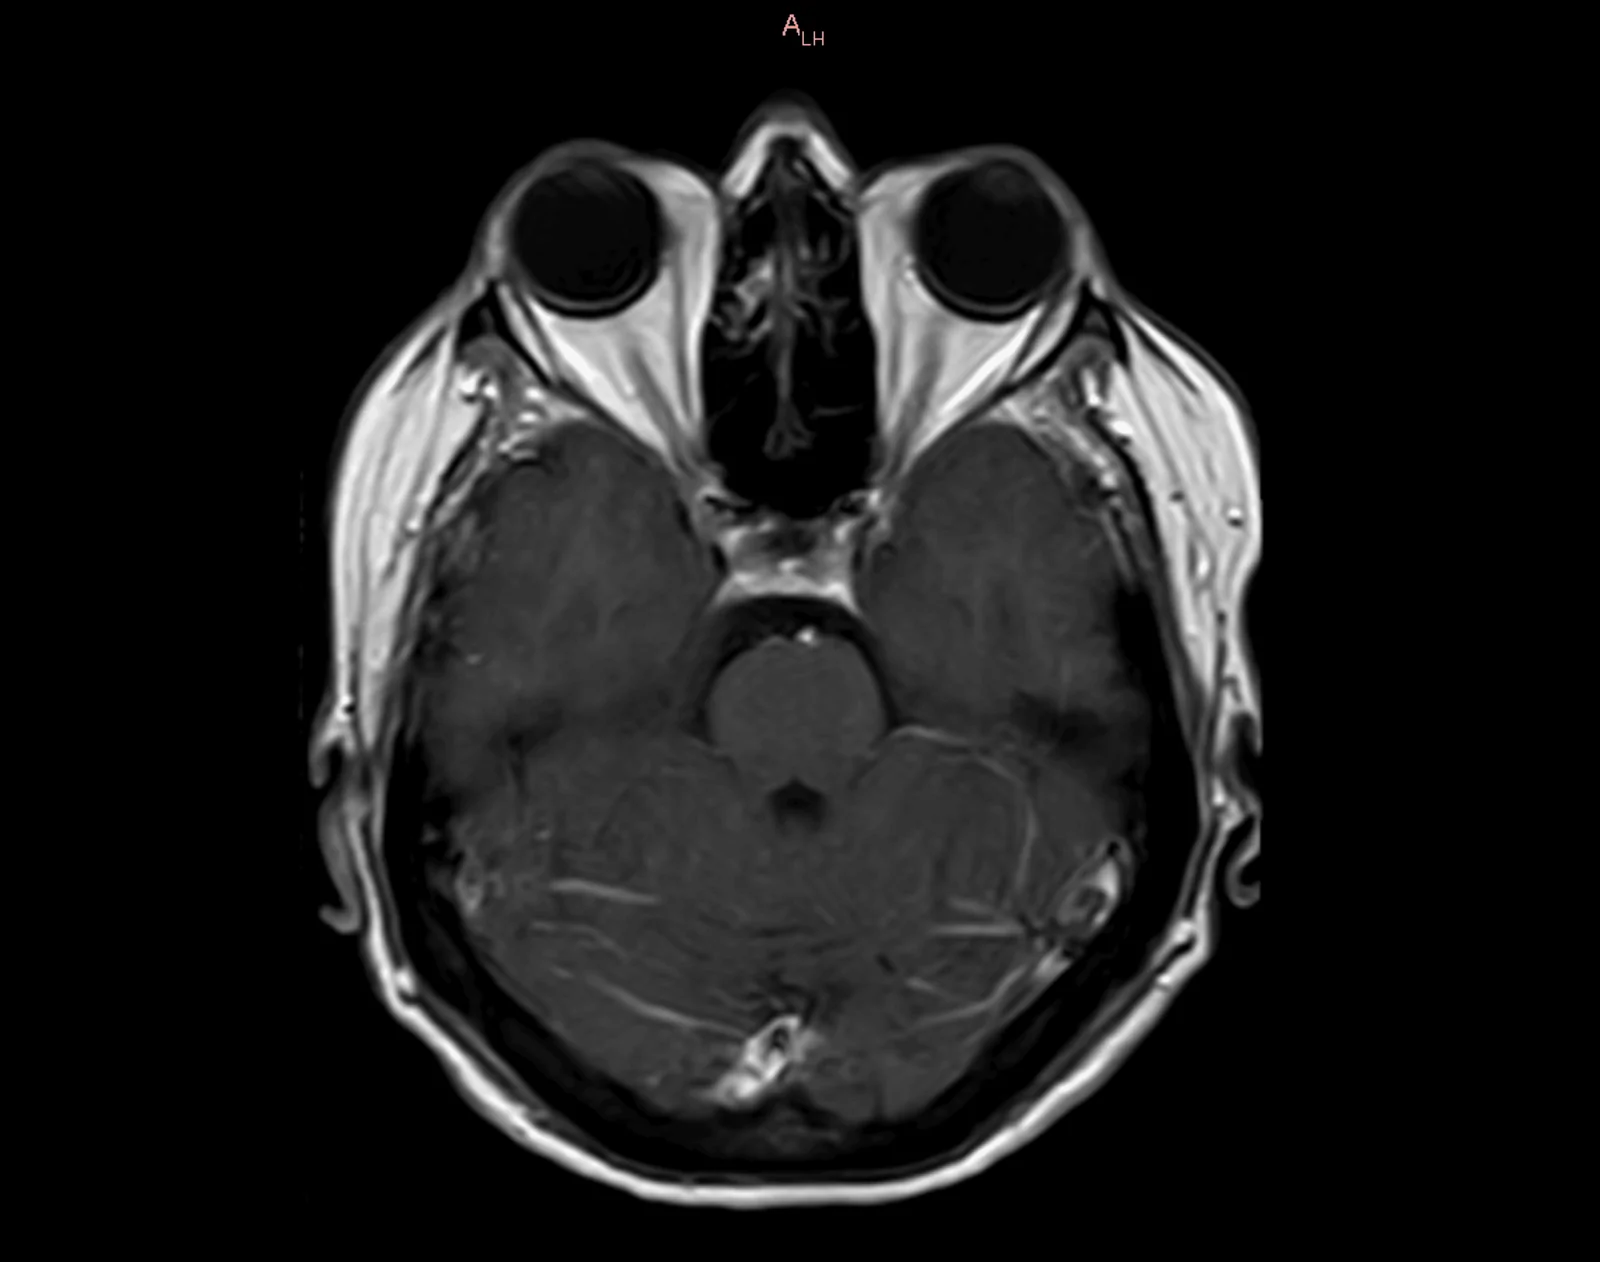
Axial post-contrast T1-weighted MRI of the brain at the level of the posterior fossa and orbits. The bilateral globes and retro-orbital structures appear intact without evidence of infiltrative disease.
